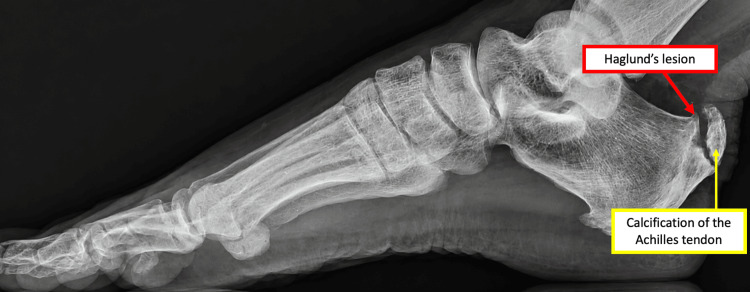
Radiographic features of Haglund syndrome Lateral foot radiograph demonstrating a prominent bony growth of the posterosuperior angle of the calcaneus and macrocalcification at the distal Achilles tendon insertion.
